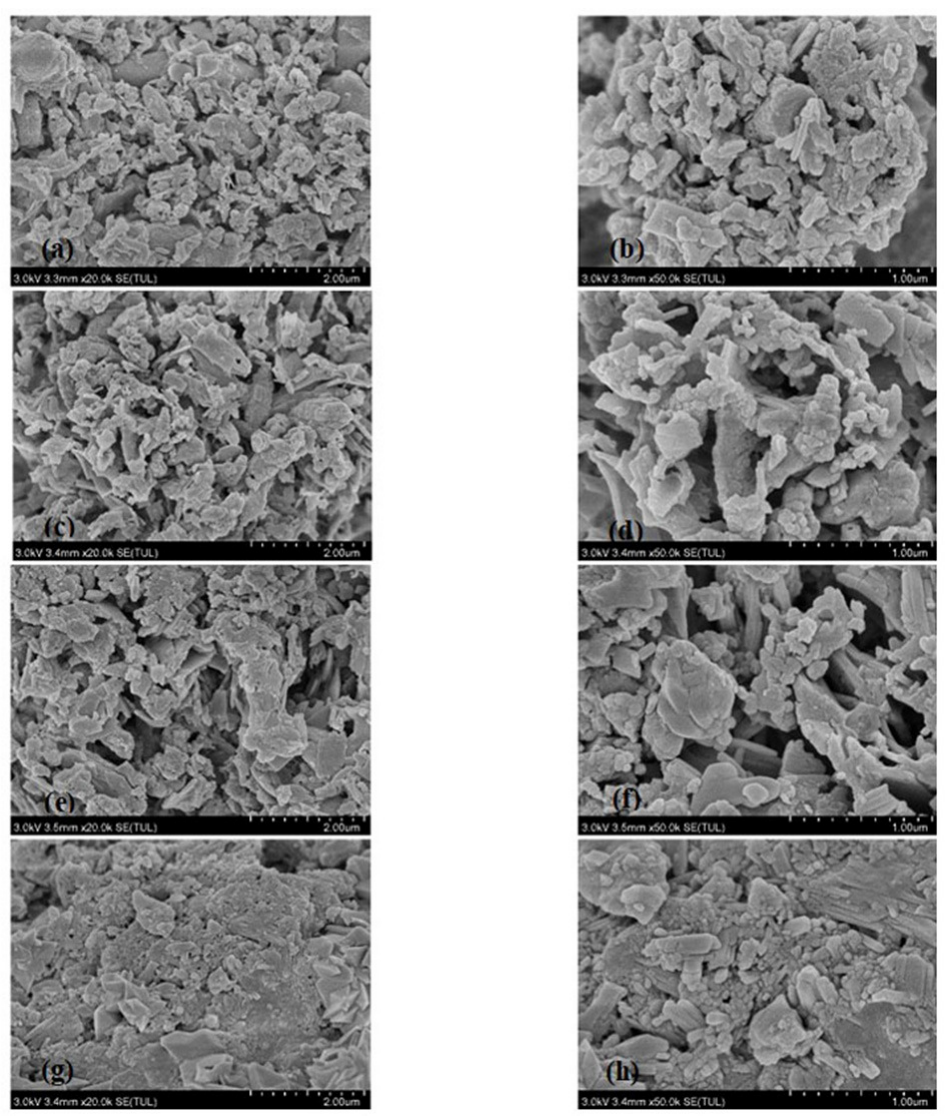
SEM images of SDF of millet bran before and after three modifications (a) untreated SDF at 20,000 ×, (b) untreated SDF at 50,000 ×, (c) high-temperature-cooked SDF at 20,000 ×, (d) high-temperature-cooked SDF at 50,000 ×, (e) high-pressure-treated SDF at 20,000 ×, (f) high-pressure-treated SDF at 20,000 ×, (g) ultrasound-treated SDF at 20,000 ×, (h) ultrasound-treated SDF at 50,000 ×.
